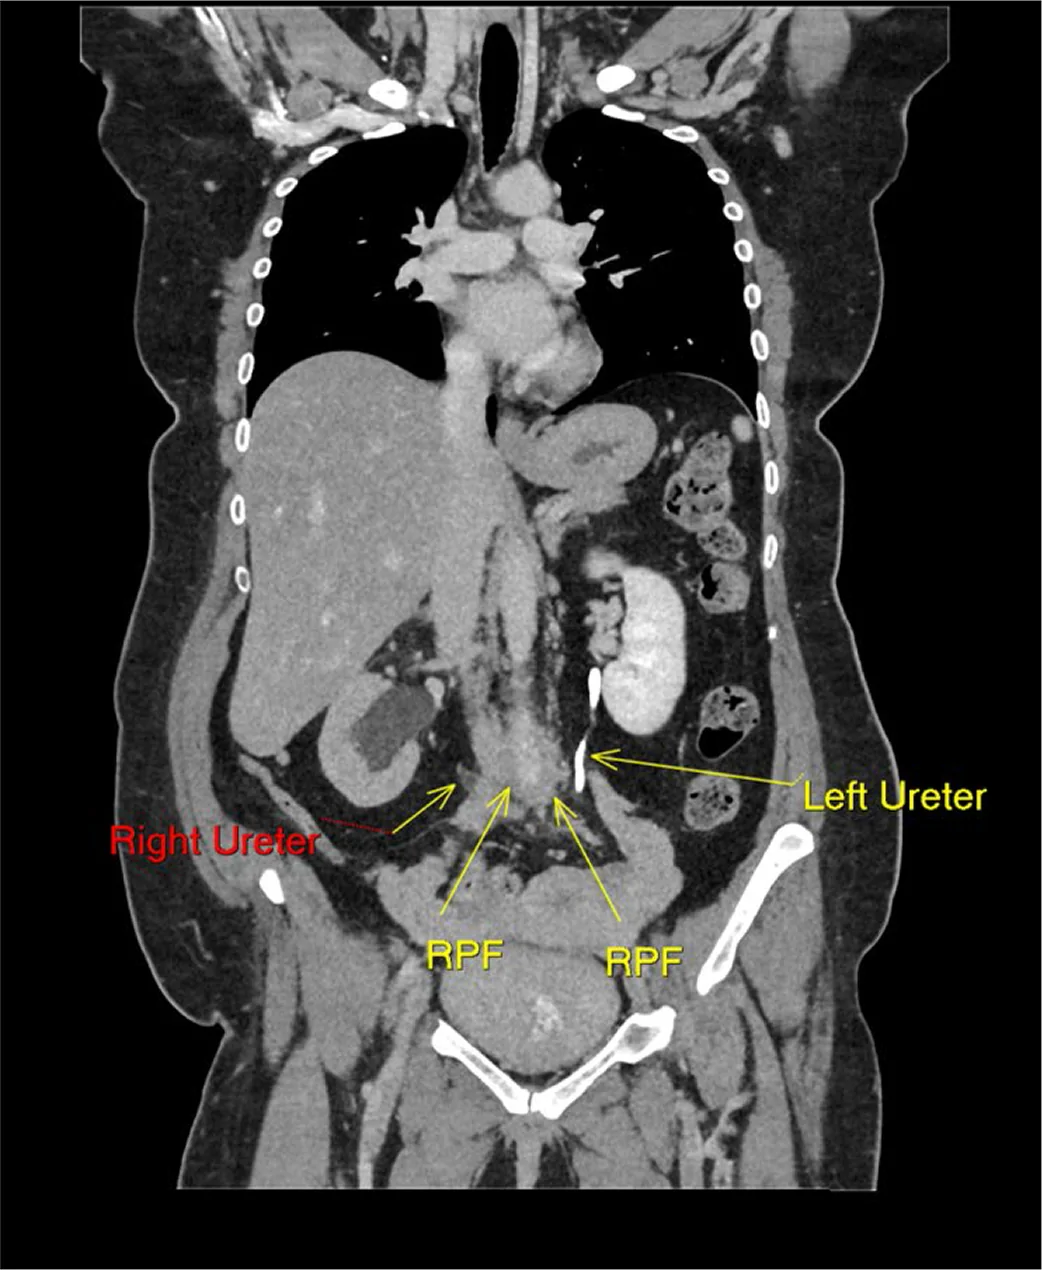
Computer Topography (CT) scan demonstrating retroperitoneal fibrosis.
